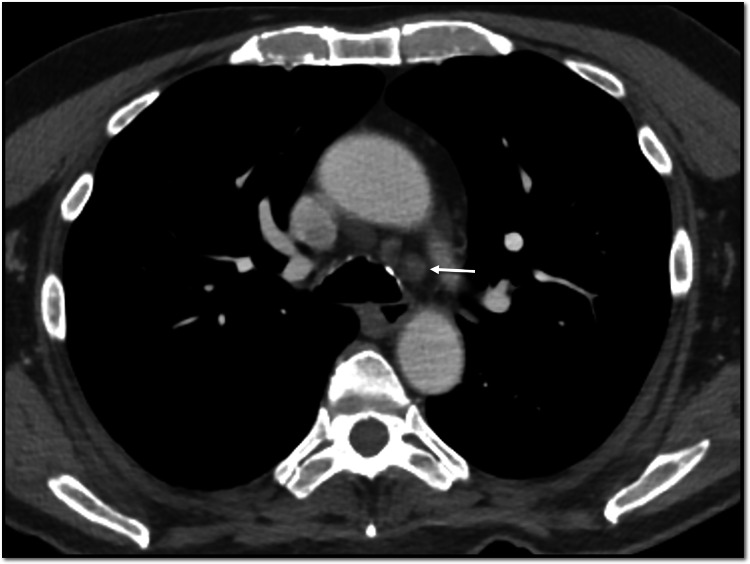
CT chest reveals mediastinal lymphadenopathy Arrow indicates low left paratracheal nodal enlargement, max 1 cm.
